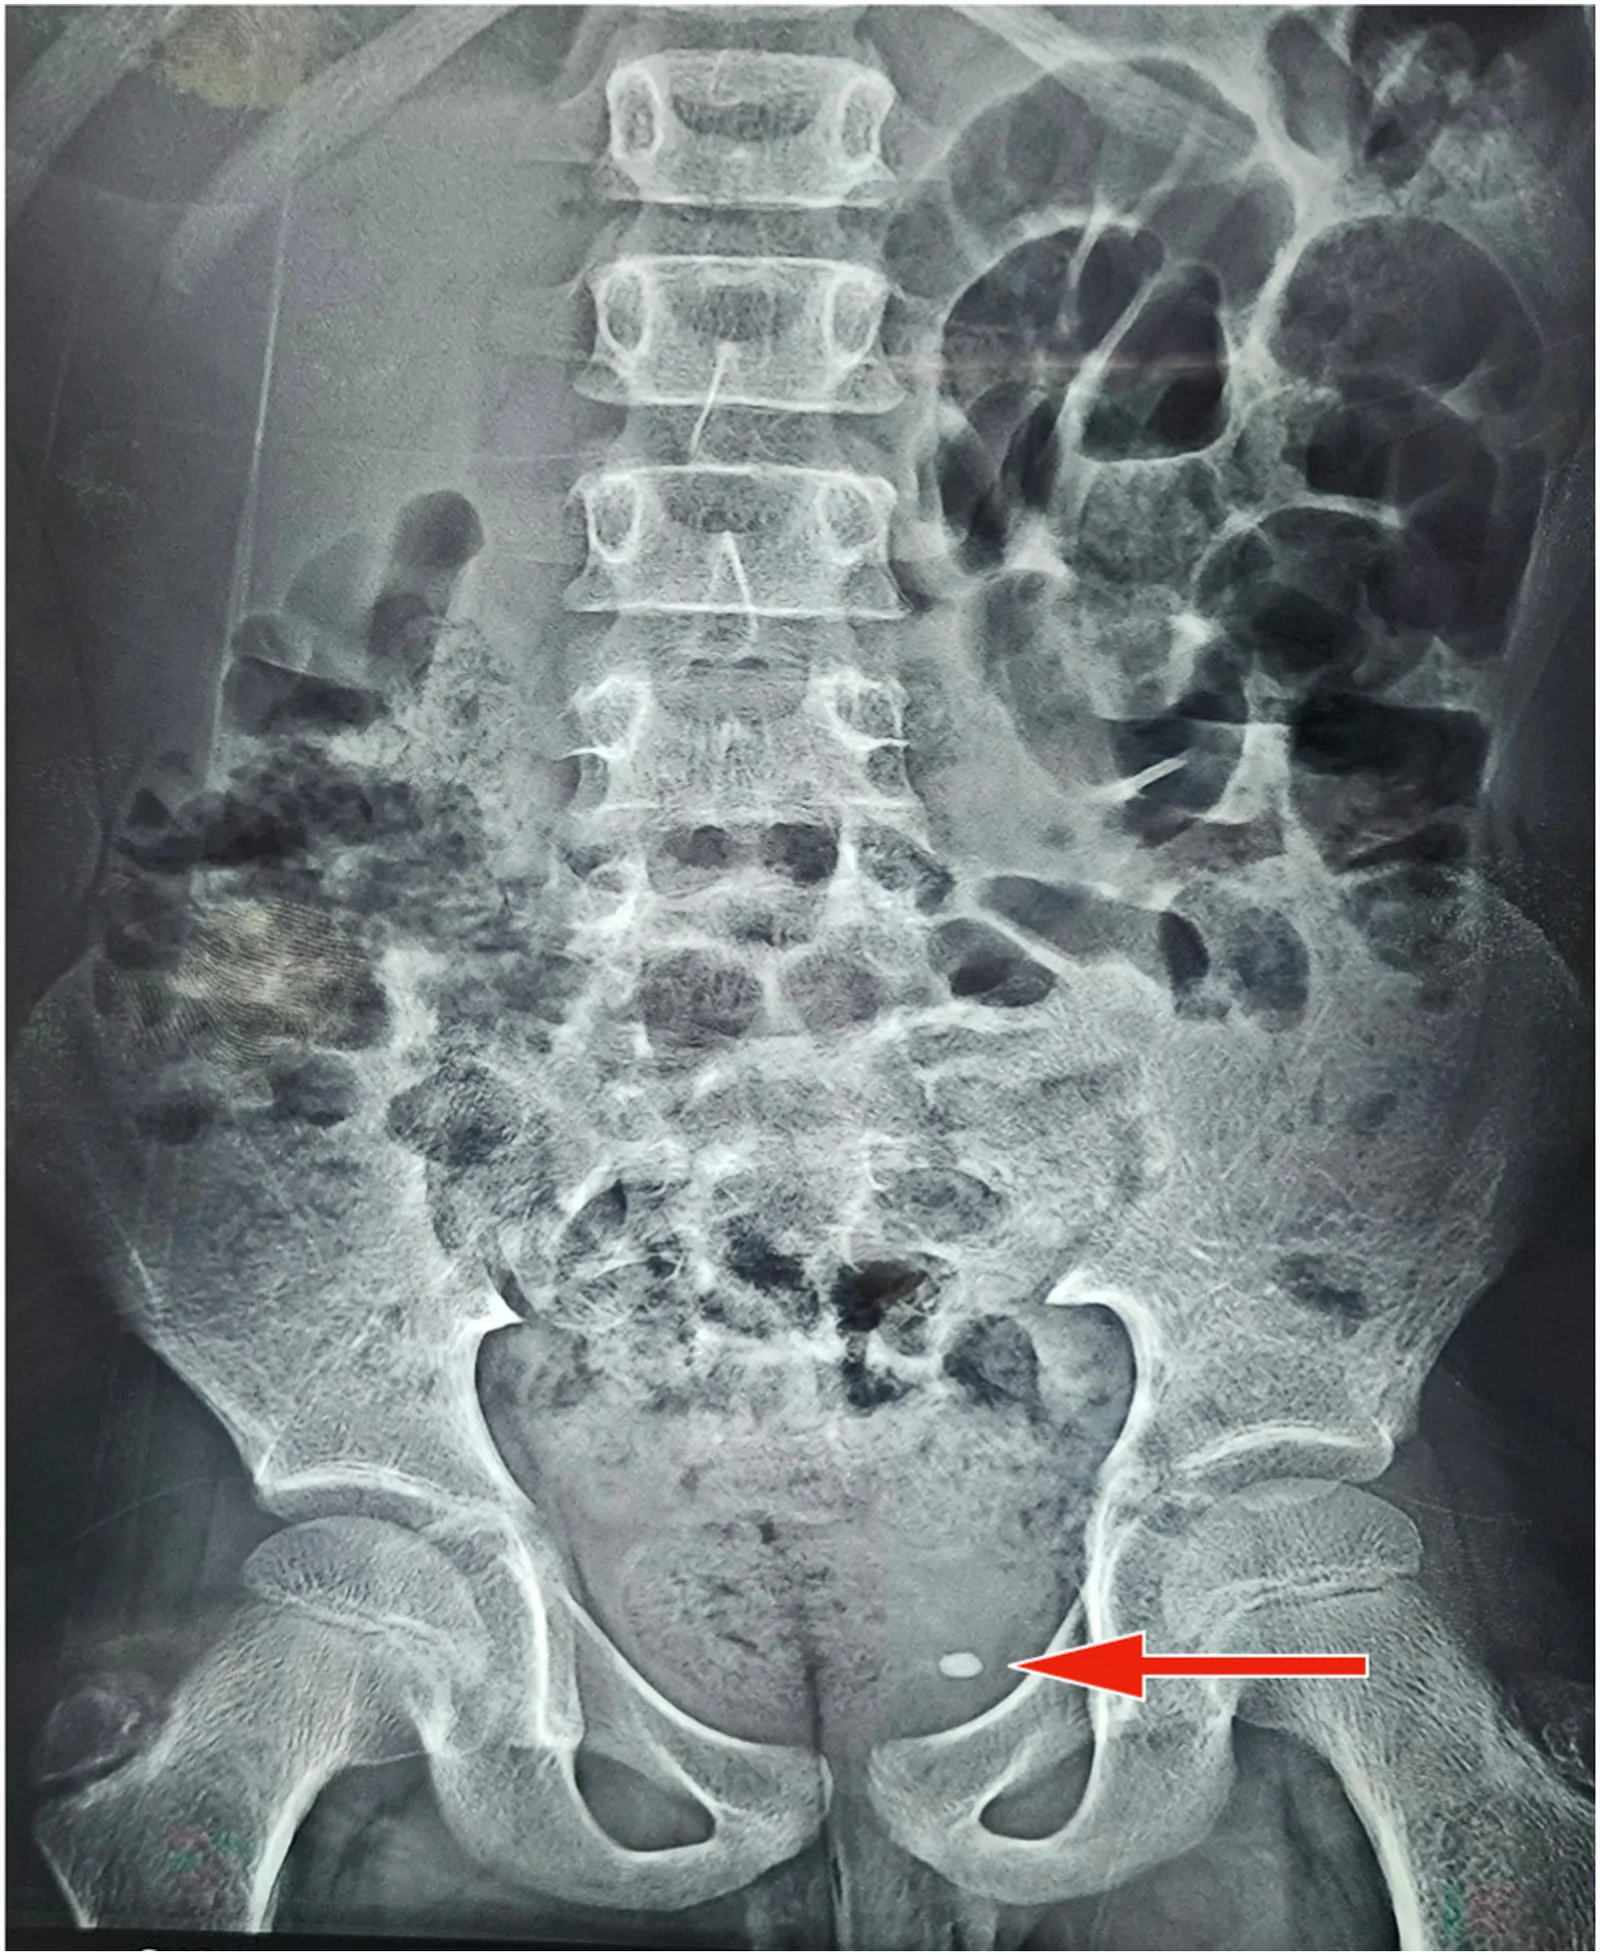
Initial plain KUB radiograph showing a solitary radio-opaque calculus projected over the left distal ureter (RED arrow).
